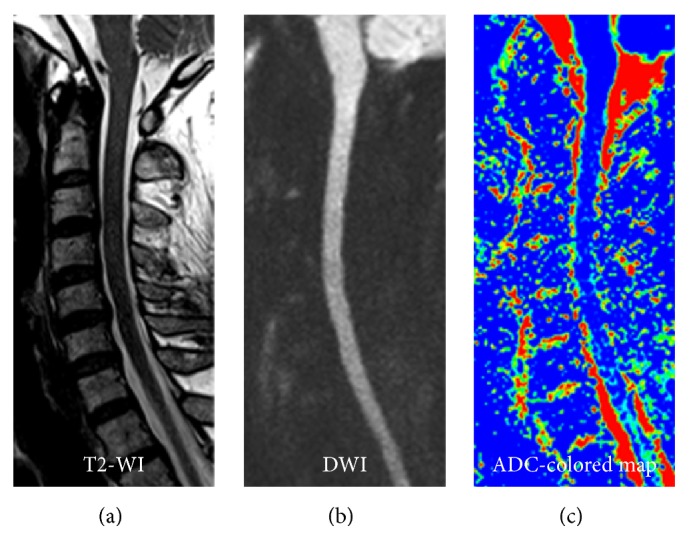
T2-WI, DWI, and ADC-colored map images of the cervical spine. (a) T2-WI image of the cervical spine, showing the anatomical information of the cervical spine and discs. (b) The diffusion-weighted image (DWI) of the cervical spine (*b* value = 0 and 500 s/mm^2^). (c) ADC-colored map of the cervical spine.
